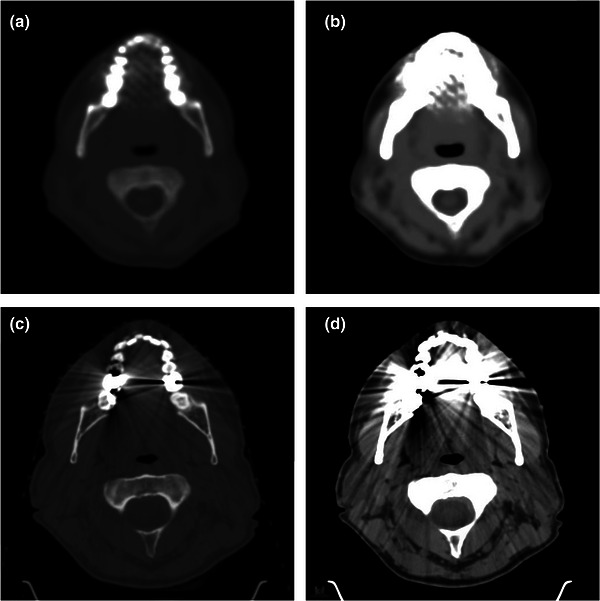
Dental implants on the synthetic‐CT in (a) bone and (b) soft‐tissue contrast windows. Implants on reference‐CT for (c) bone and (d) soft‐tissue windows. Streaking artifacts are eliminated in the sCT, and artifacts instead are more localized to the bones.
